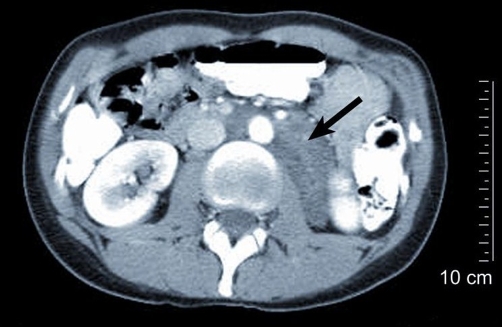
CT scan of the abdomen showing a retroperitoneal mass (arrow).
